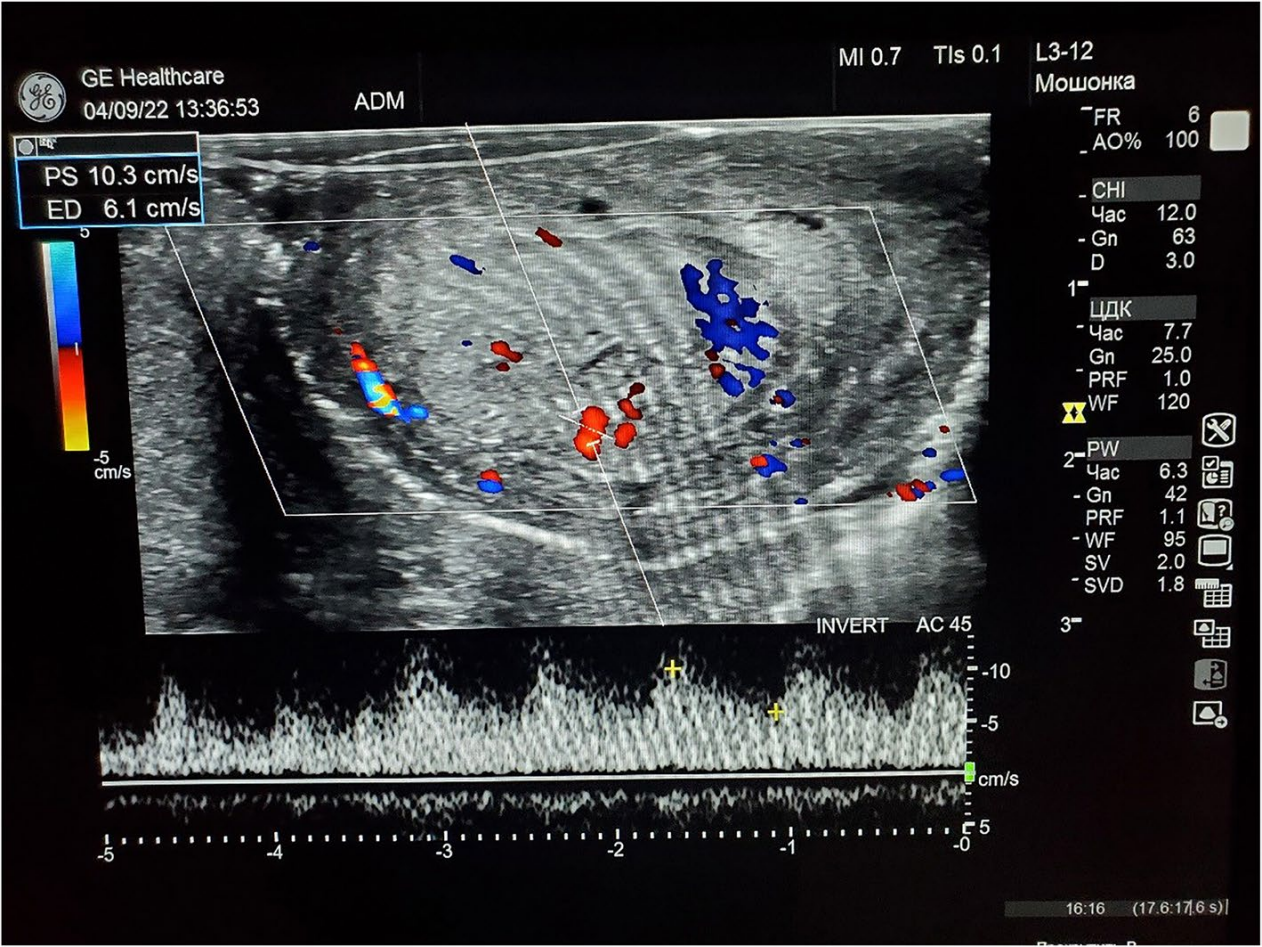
Doppler ultrasonography of the arteries of the penis: determines the main blood flow in the arteries of the corpora cavernosa (MSC 16–24 cm/s, Ri 0.73–0.77); in the head small arteries with collateral blood flow MSC 0.7 ml/s, Ri 0.62
